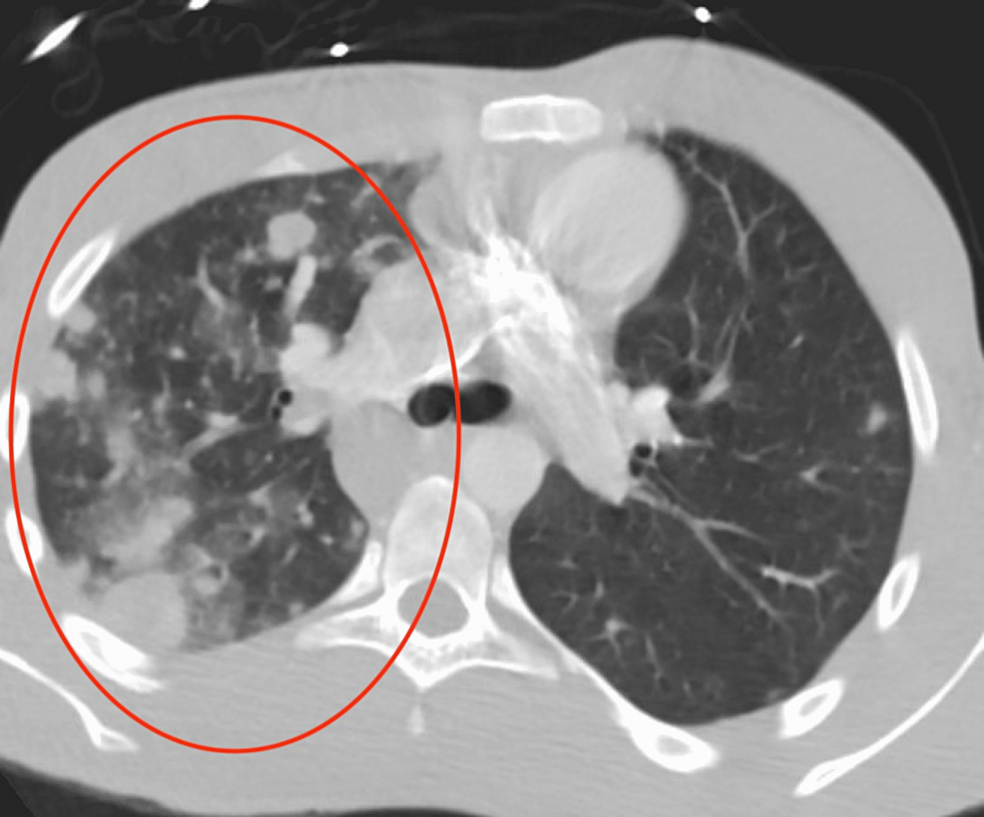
Initial thoracic CT. Red circle showing numerous nodules in the lung
